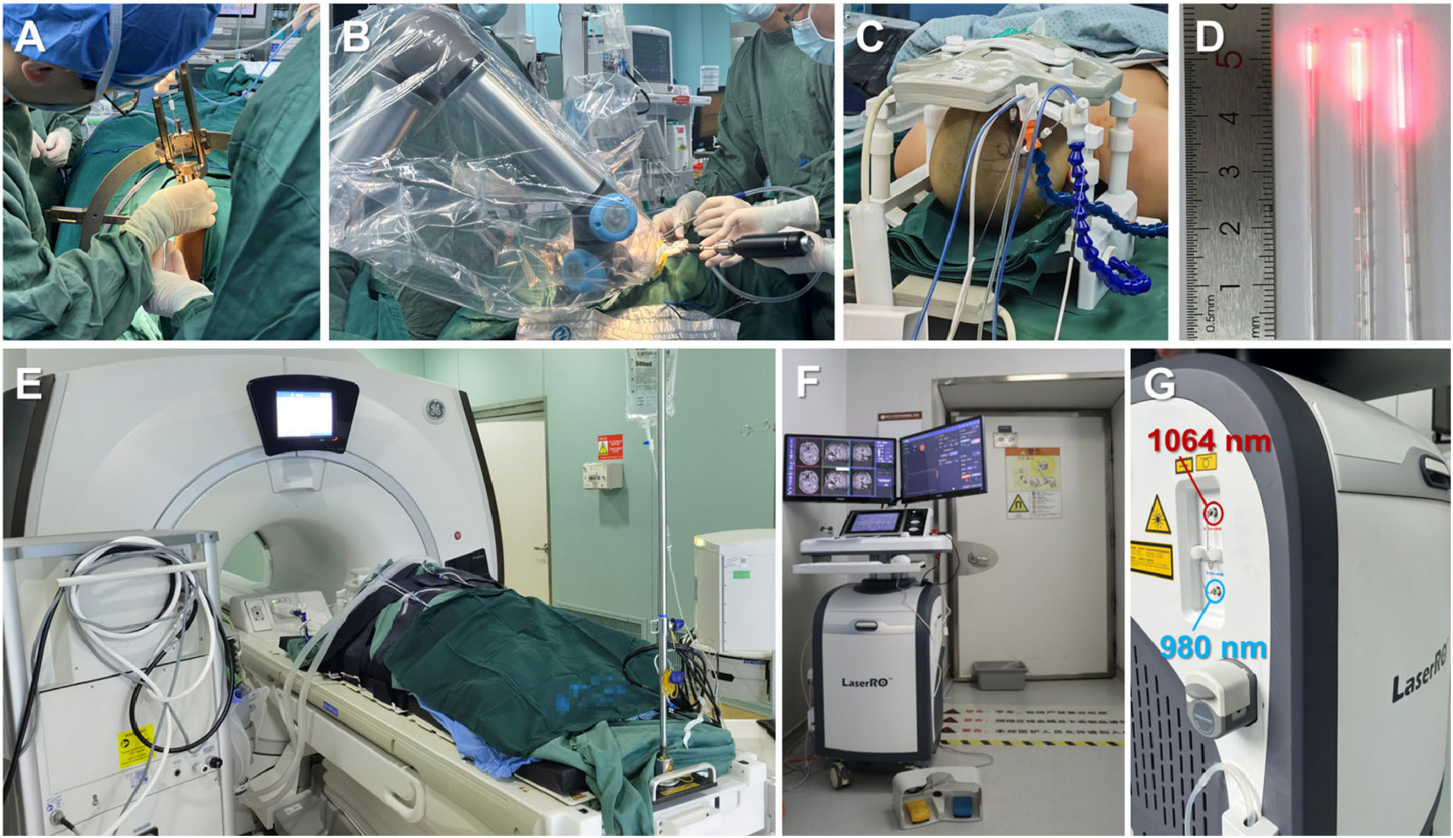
LITT procedure was performed in the intraoperative MRI room. (A) Optical fiber implantation under Leksell frame; (B) neurosurgical robot assisted optical fiber insertion; (C) intraoperative MR coil fixation after optical fiber insertion; (D) Fiber‐optic catheter outer (length 4, 10, 15 mm); (E) patients in the intraoperative MRI room; (F, G) the LaserRO system equipped with a dual‐wavelength laser, operating at 980 and 1064 nm, for MRgLITT.
